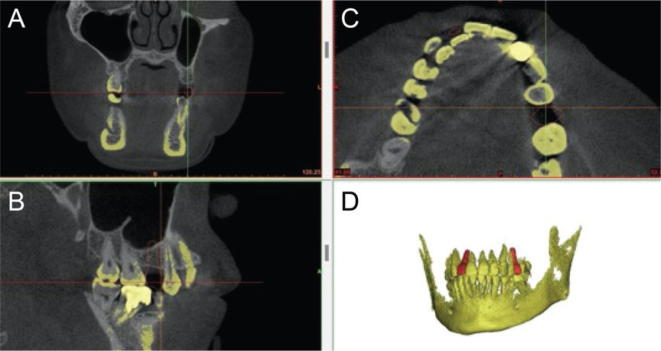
Alignment of CBCT images before implantation and 3 years after implant loading, with the yellow portion in the images representing CBCT images three years after implant loading. Figure 1A is a coronal section screenshot of the aligned images, Figure 1B is a horizontal section screenshot of the aligned images, Figure 1C is a sagittal section screenshot of the aligned images, and Figure 1D is a 3D screenshot of the aligned images.
